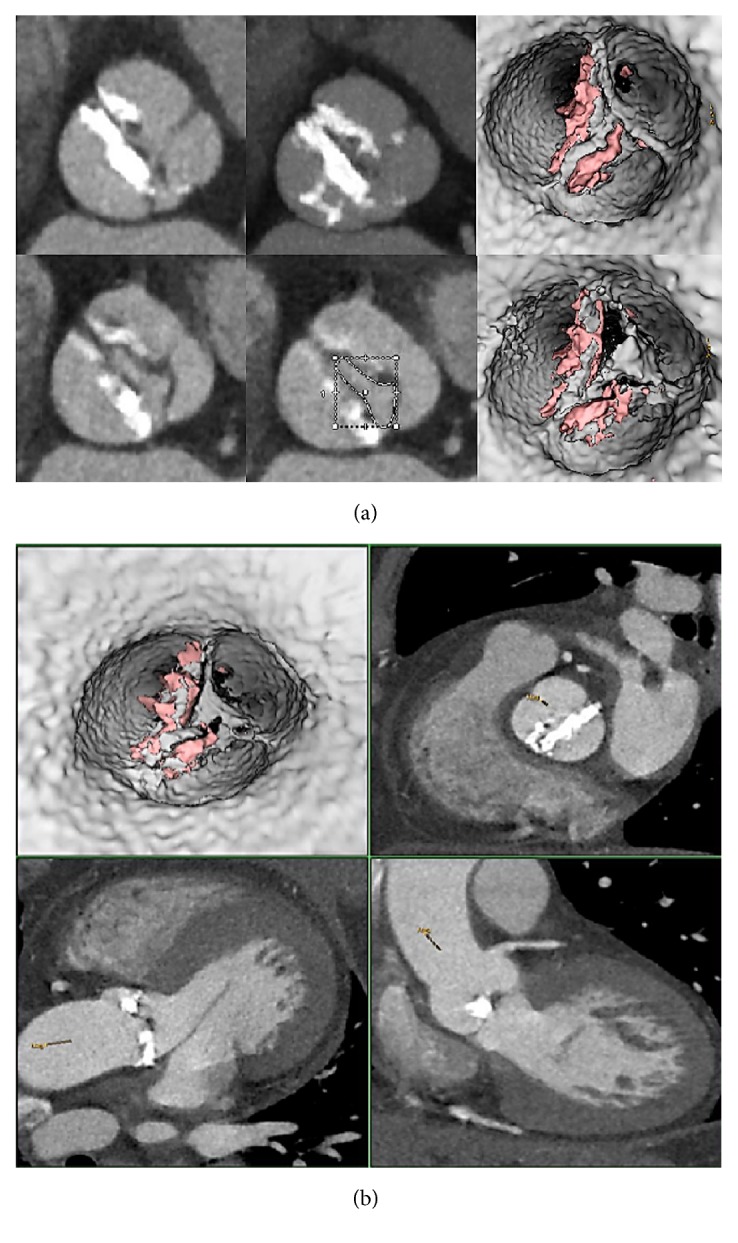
320 CT scan shows refractory calcifications on aortic leaflets of a prohibitive high-risk patient who undergo TAVR. The procedure performed was as follows: one beat full cardiac cycle (0-100%) acquisition DLP = 459 mGy/cm for functional aortic valve assessment, morphological aortic valve study, and anatomical AVA determination.
